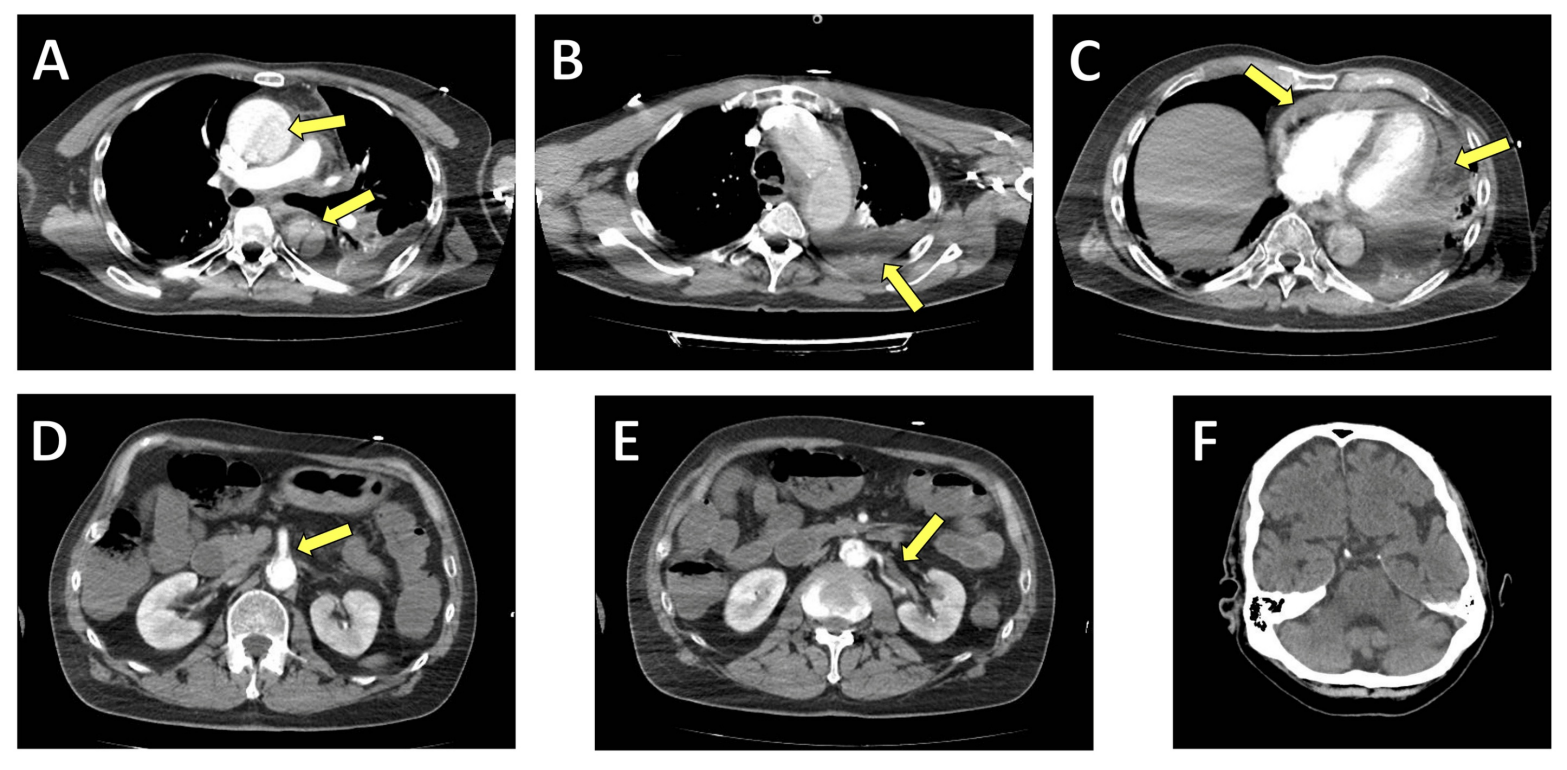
Preoperative imaging findings: A) Acute type A aortic dissection with a dissected ascending aorta (arrow). (B) Left pleural effusion (arrow). (C) Pericardial effusion (arrow) caused by endopericardial rupture of the aorta. (D) Abdominal CT scan showing dissection extending into the abdominal aorta, with involvement of the superior mesenteric artery (arrow). (E) Dissection of the renal arteries. (F) Preoperative brain CT scan performed as a baseline due to cardiac arrest and the uncertain prognosis for postoperative neurological recovery.
